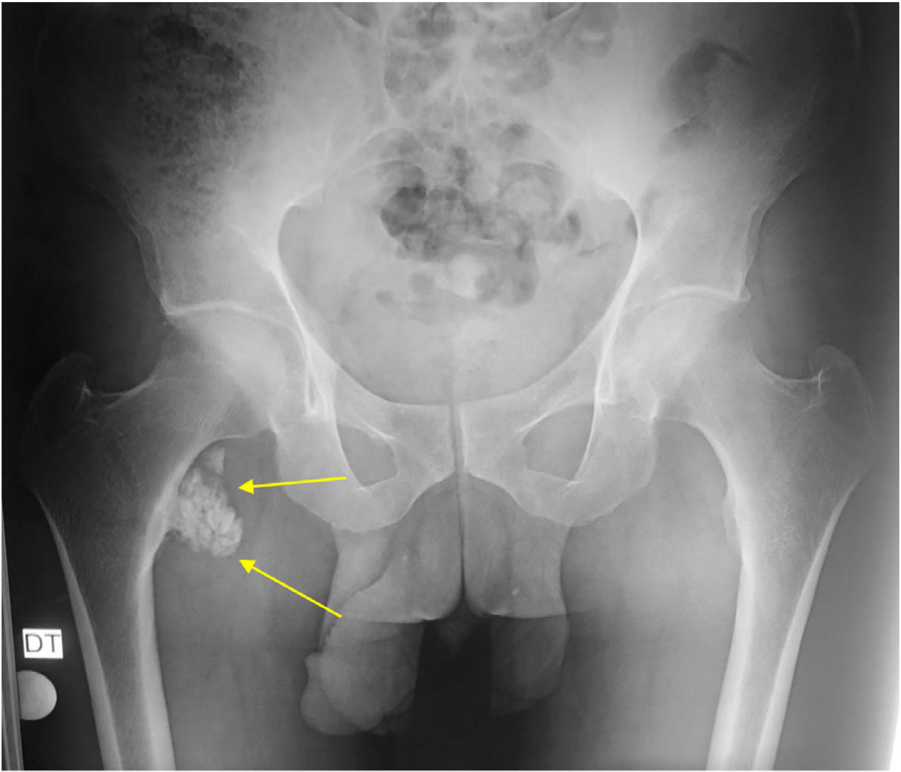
The plain radiographs of the pelvis reveals multiple high-density opacities consistent with soft-tissue calcifications opposite the left greater trochanter without osseous lesion (yellow arrows).
